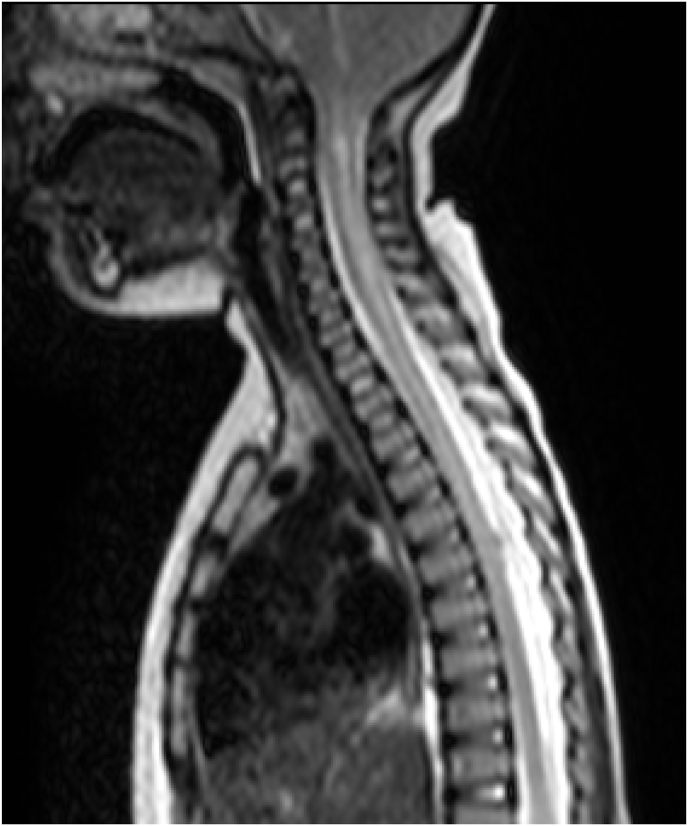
Gadolinium-enhanced magnetic resonance imaging of the whole spine was done and revealed extensive diffuse hyper intense signal involving predominantly the grey matter of the upper cervical spinal cord. Mild enlargement and swelling of the cervical cord were also noted.
